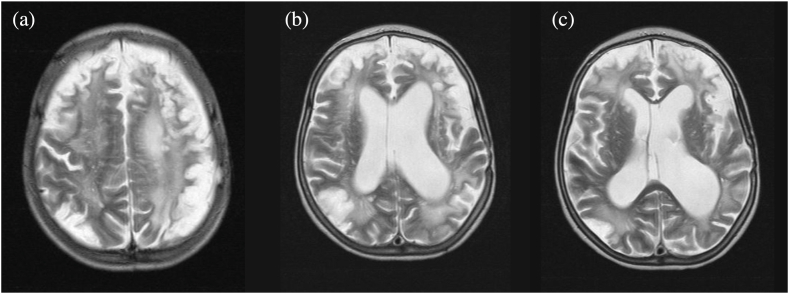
T2-weighted MRI taken(a) below the level of the caudate nucleus, (b) at the level of the caudate nucleus and genu of the corpus collosum, and (c) at the level of the occipital horns of the lateral ventricles, after the patient presented with her third stroke. This MRI shows subacute infarction in almost the entire right temporal lobe corresponding to MCA territory, with increased diffusion without significant mass effect. There are similar findings in the cortical gray matter anteriorly in left temporal lobe with increased diffusion. There are severe old ischemic lesions bilaterally, more on the left side, which are unchanged since the previous study with secondary enlargement of the ventricular system supratentorially. The posterior fossa is grossly unremarkable.
